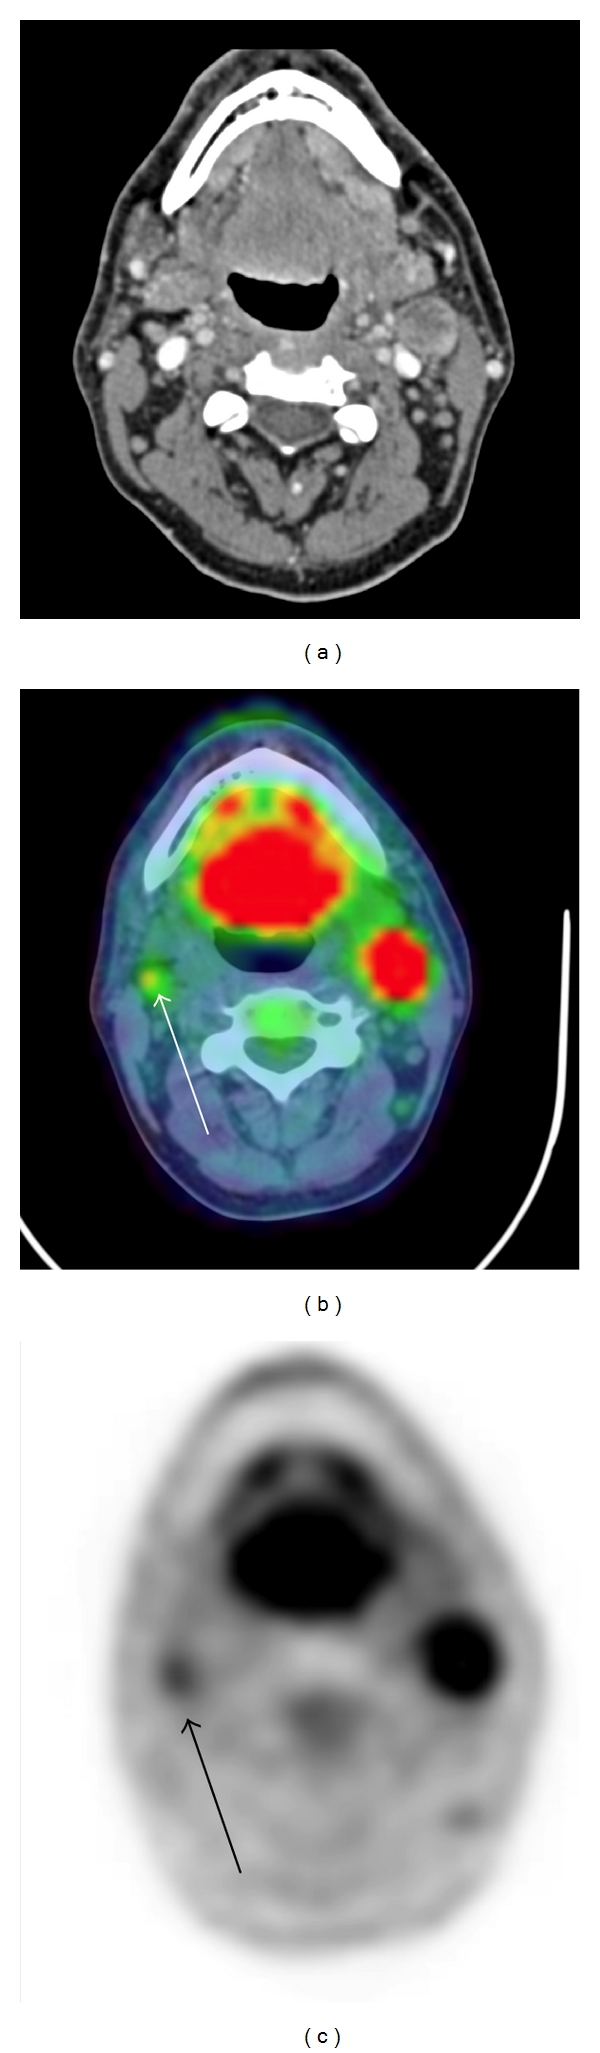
Altered TNM staging and lymph node level identification with FDG PET-CT. T4 squamous cell carcinoma of left tonsil. Diagnostic CT identified T4 N2b disease. FDG PET-CT identified a subcentimetre (SUV_max_ = 3.5) contralateral lymph node, altering staging to T4 N2c. (a) Diagnostic contrast-enhanced CT. (b) Coregistered FDG PET-CT. (c) FDG PET. White arrow = right level II lymph node.
